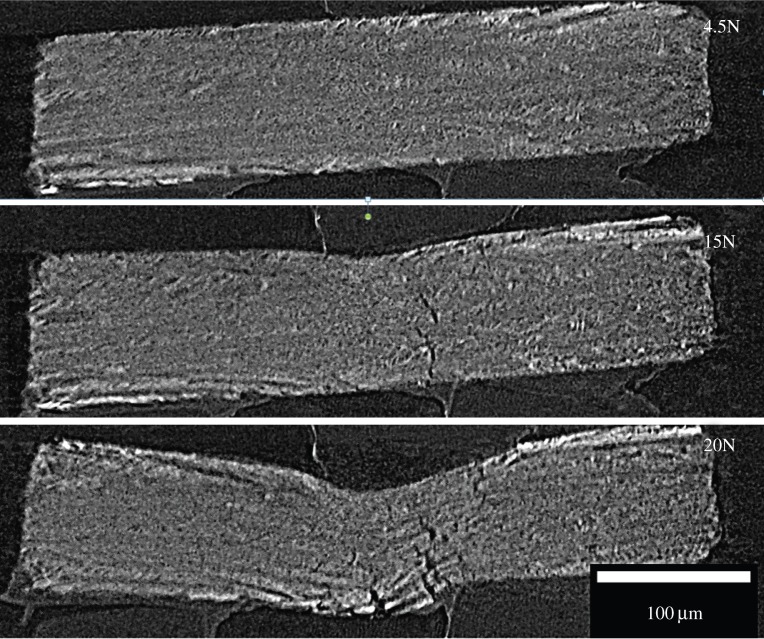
Time-lapse synchrotron X-ray tomographic cross-sections (I13 Diamond Light Source) showing crack deflection in a freeze cast layered ceramic (approx. 4×0.8×1.6 mm) fractured in bending. (Courtesy of D Eastwood, E. D'Elia, E. Saiz. S Yue, C. Rau, P.J. Withers and P. D. Lee.)
